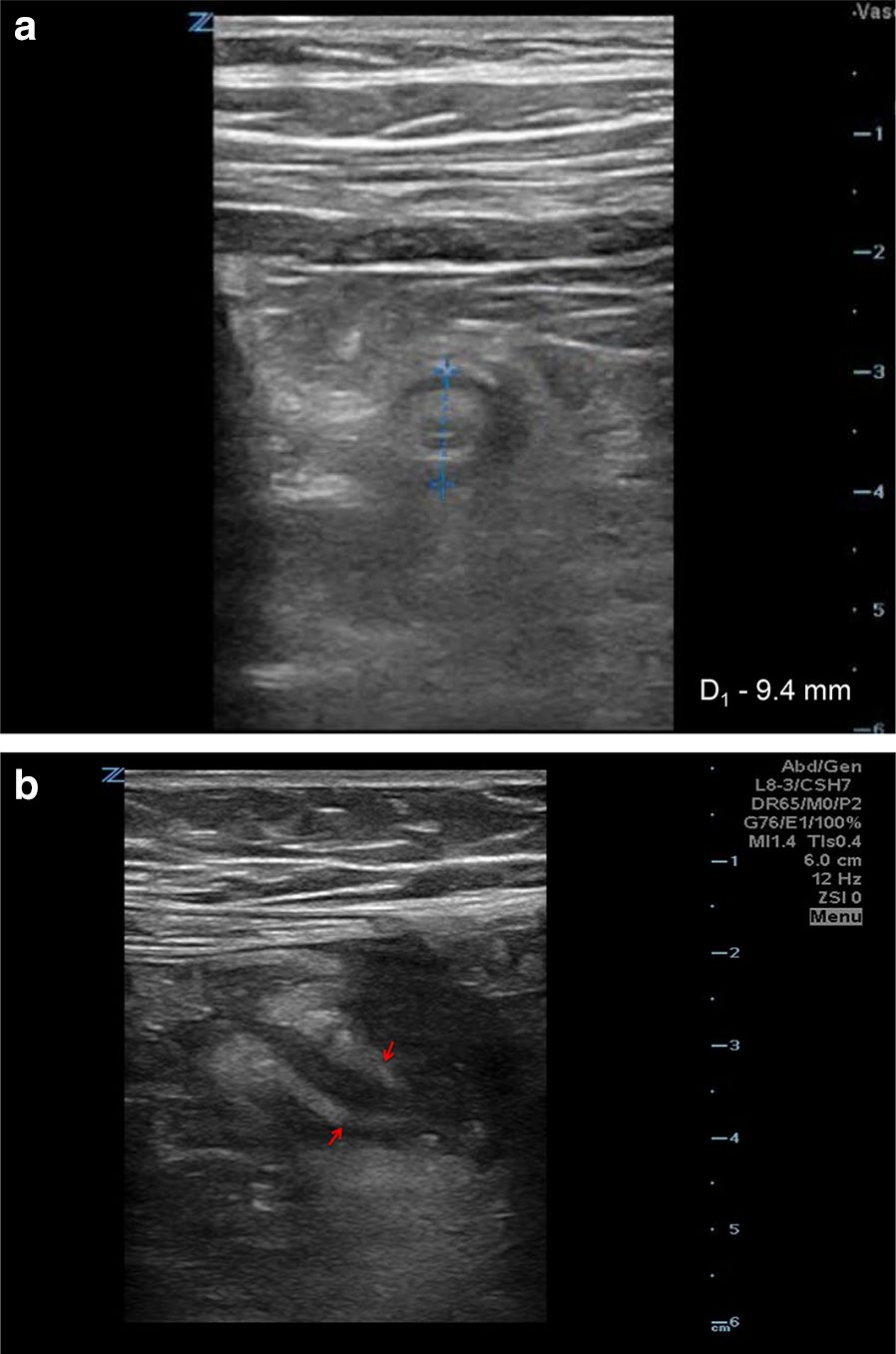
a Transverse (short axis) ultrasound scan shows SA measuring 9.4 mm in diameter. b Longitudinal ultrasound scan shows tubular blind-ended structure with surrounding with free fluid
